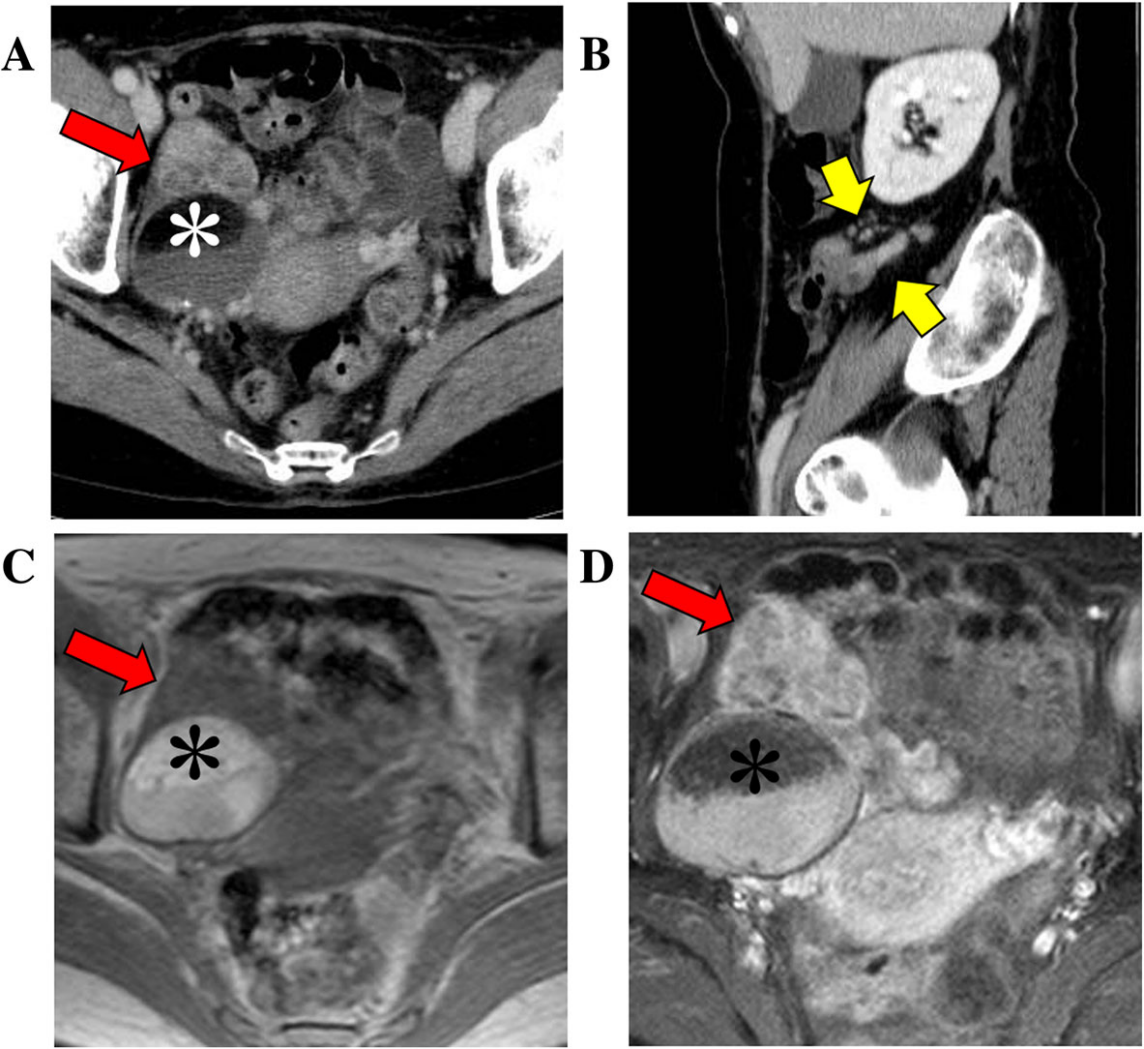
Clinical imaging. Enhanced computed tomography reveals the presence of: a a 70-mm right ovarian mass with a 40-mm round mass with fat (white asterisk) and solid component with non-uniform enhancement at the ventral side (red arrow) and (b) a 10-mm enlarged appendix with strong enhancement (yellow arrows). Magnetic resonance imaging of (c) T1-weighted image and (d) Fat-saturated T1-weighted image with gadolinium reveals fat (black asterisks), water, and solid components (red arrows)
